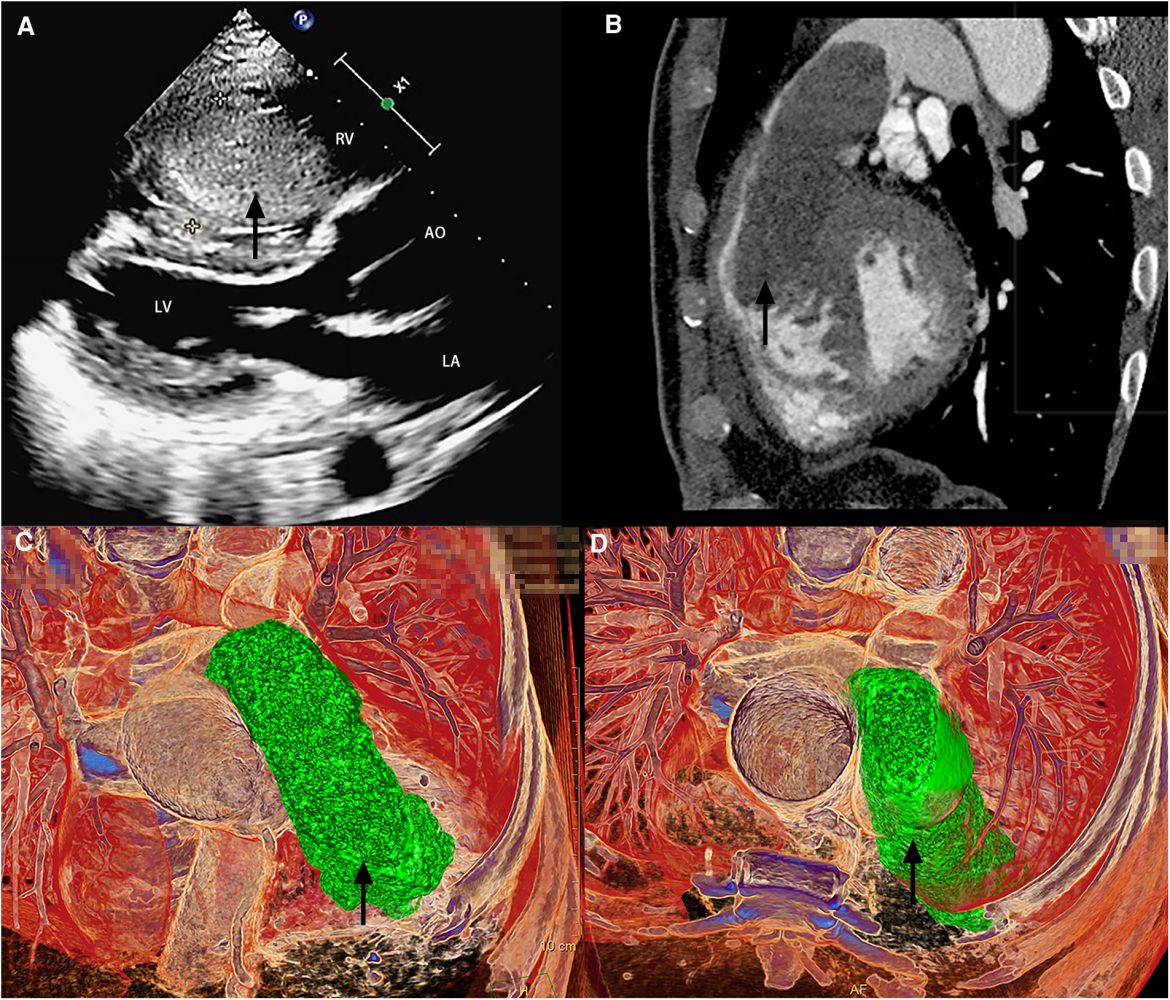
Imaging examination. Transthoracic echocardiography (A) and cardiac computed tomographic angiography (B–D) revealed a mass located within the right ventricle (black arrow). RV, right ventricle; LA, left atrium; LV, left ventricle; and AO, aorta.
